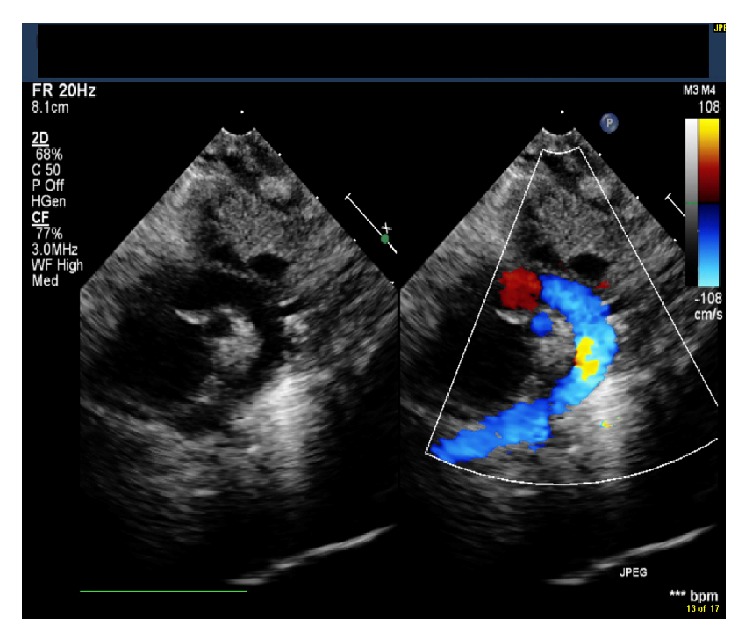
Echocardiogram (suprasternal view) demonstrating a normal aortic arch without any evidence of coarctation prior to patent ductus arteriosus ligation surgery.
